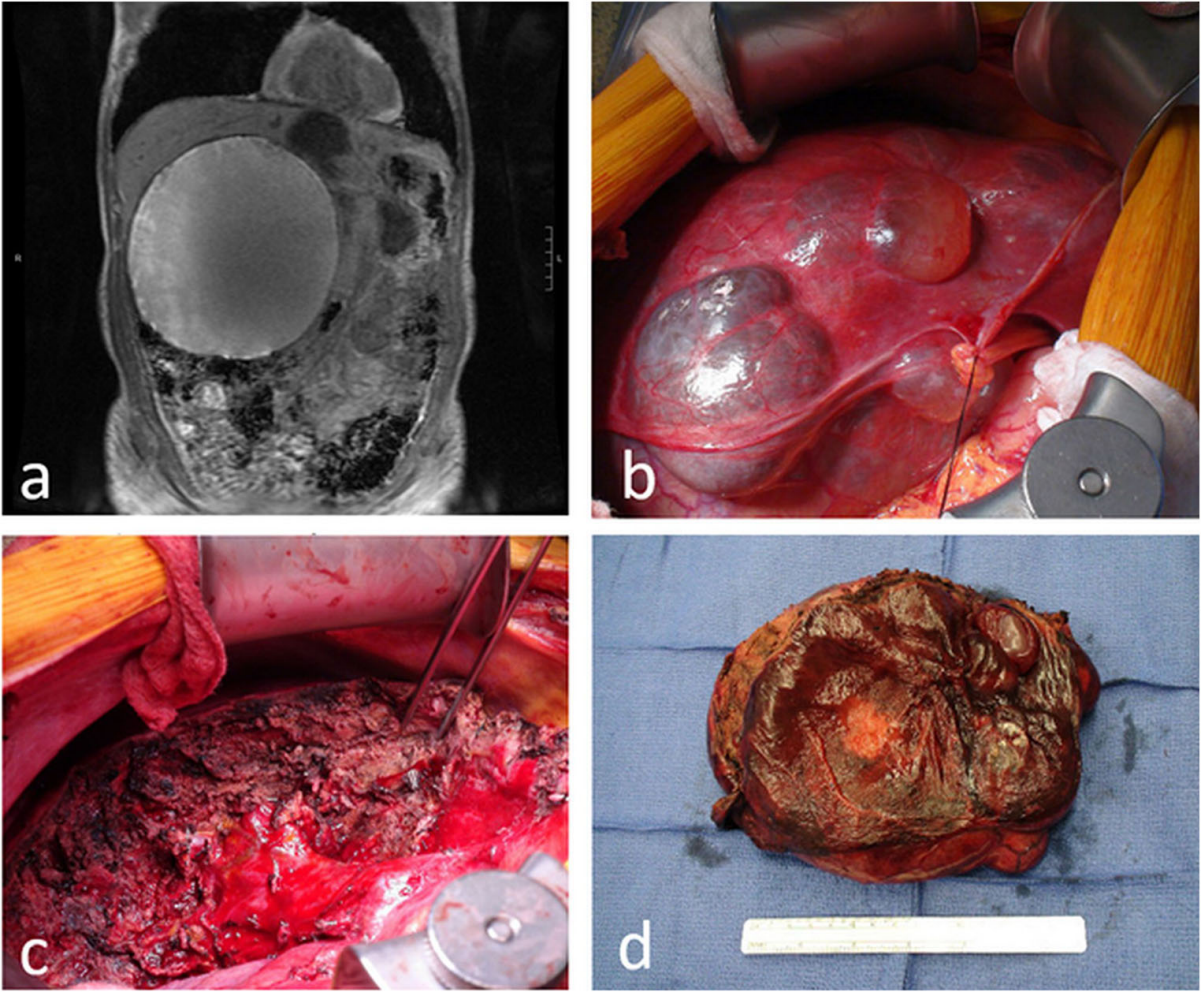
a Coronal magnetic resonance image of a 19-cm cyst in a 70-year-old man with features concerning for mucinous cystadenoma. b Anterior surface of the liver during open approach. c Transection line for partial segment IVa and IVb hepatectomy. d The cyst removed intact, which resulted with benign pathology
